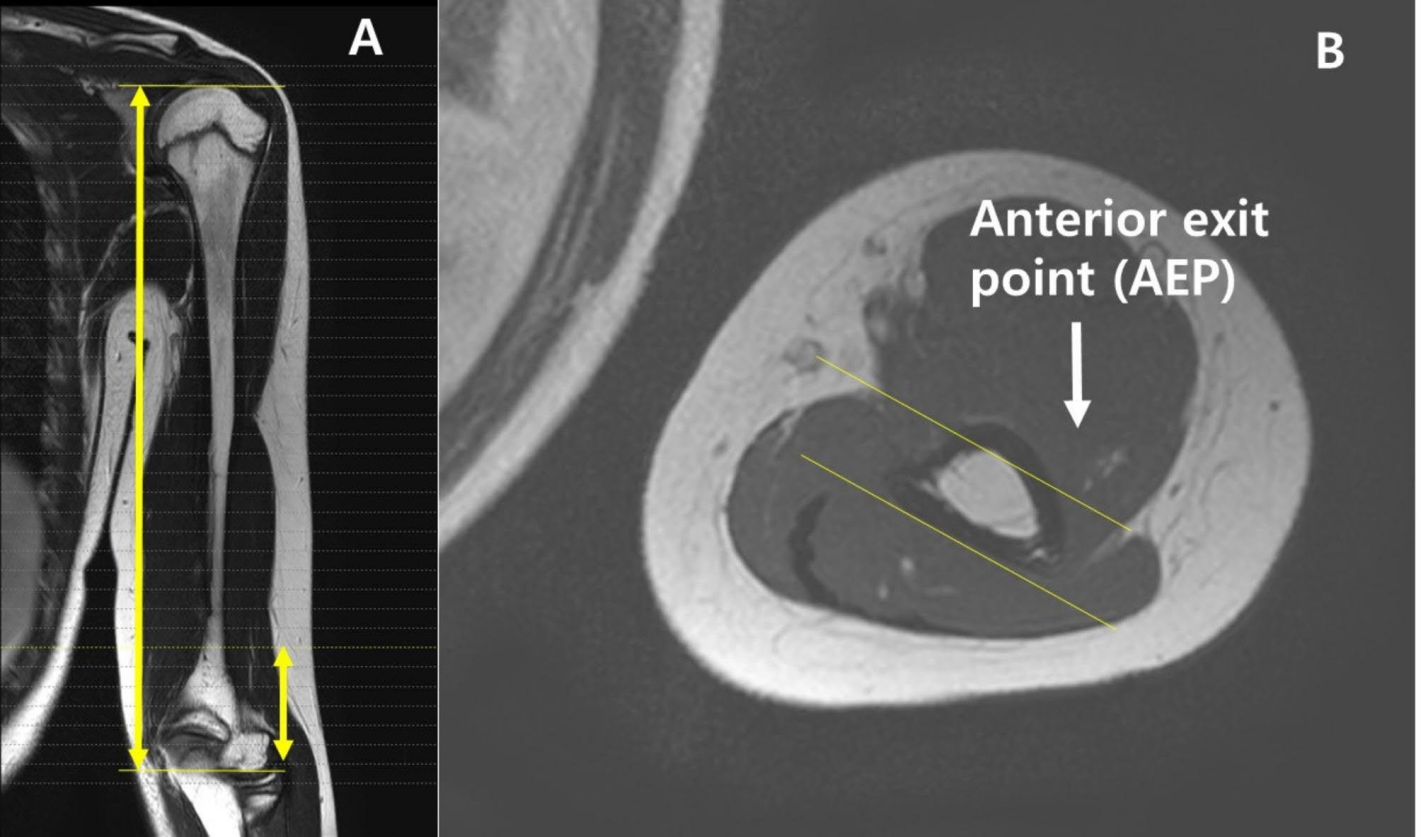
Defining landmarks for radiographic measurement. (a) The proximal landmark for measuring humerus length was set as the highest protruding point of the humeral head. The distal landmark for measuring humerus length was set as the lowermost margin of ossified bone of the lateral condyle. For children or adolescents with incomplete ossification, the landmark for measuring was set as the lowermost ossified margin of the ossification center of the capitellum. (b) The point where the nerve leaves the lateral intermuscular septum into the anterior humerus was designated as the anterior exit point (AE)
